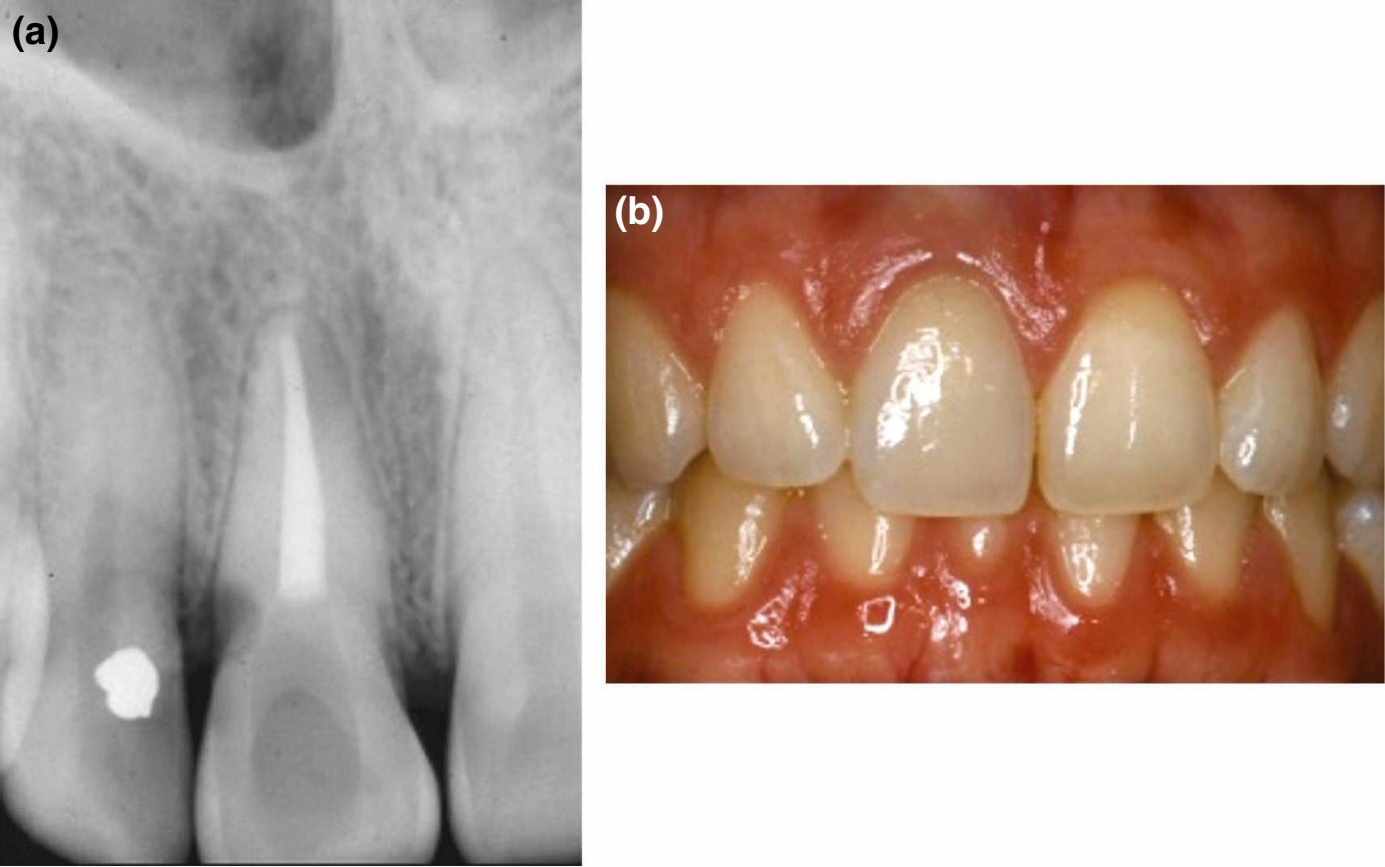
(a) A clinical photograph of the bleached left maxillary central incisor that had been bleached with the walking bleach technique with unknown medicaments. (b) A periapical radiograph revealing external cervical root resorption. Note the absence of any intracoronal barrier and large access cavity preparation. Courtesy of Professor Geoffrey Heithersay
